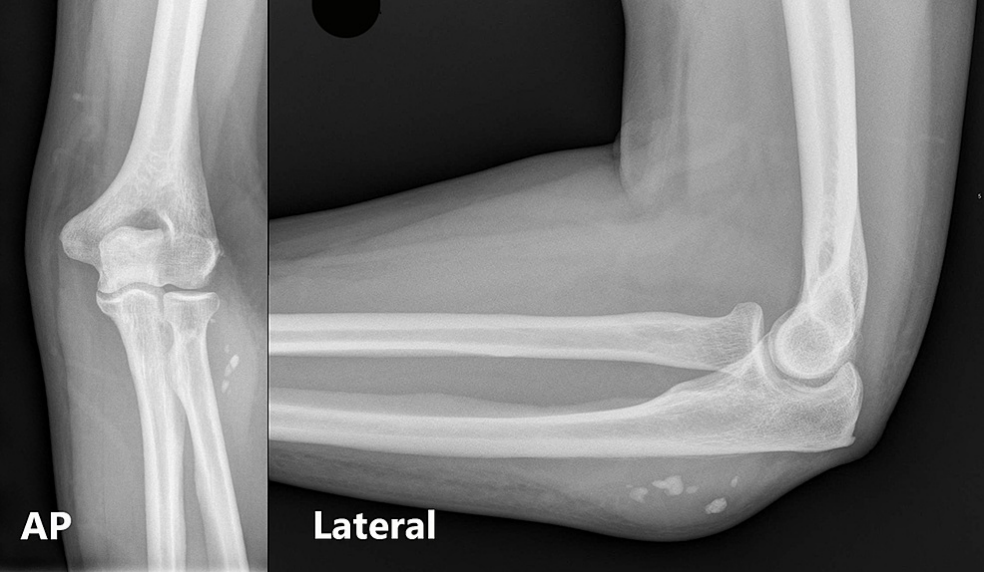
X-rays of the elbow on initial presentation with 'foreign bodies' present
